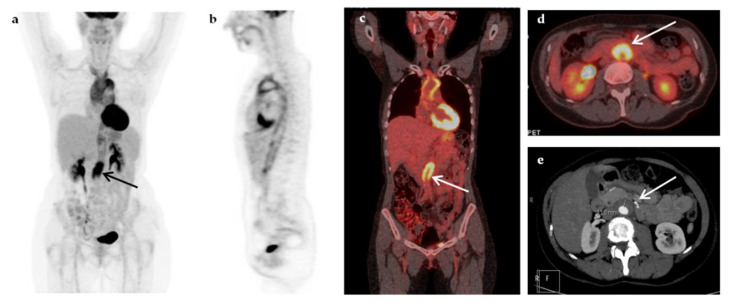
A 52-year-old woman presented with prolonged fever (over 6 months) and increased inflammatory blood markers (ESR 76 mm/h, CRP 9.3 mg/dL). The patient had a medical history of breast cancer seven years ago. Maximum-intensity projection ^18^F-FDG-PET (a), sagittal ^18^F-FDG-PET (b), coronal fused ^18^F-FDG-PET/CT (c), and transaxial fused FDG-PET/CT images at the level of the renal vessels (d) demonstrated increased metabolic activity within the wall of the thoracic and abdominal aorta, extending to the subclavian arteries, more intense at the root of the aorta and above the aortic bifurcation (arrows). The findings were compatible with vasculitis of large- and medium-sized arteries, mainly affecting the aorta (panaortitis). Transverse section of the CT angiography at the level of renal vessels (e) showed thickening of the aorta wall, more pronounced at the level below the renal vessels (arrow), but with no evidence of narrowing of the aortic lumen. A diagnosis of large vessel vasculitis/Takayasu’s arteritis was made, and fever resolved after treatment with corticosteroids.
